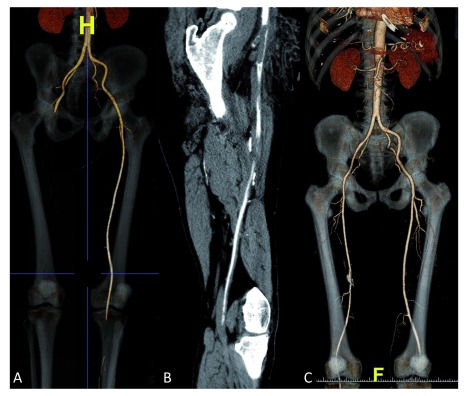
Lower limb contrast enhanced computed tomography images. (
